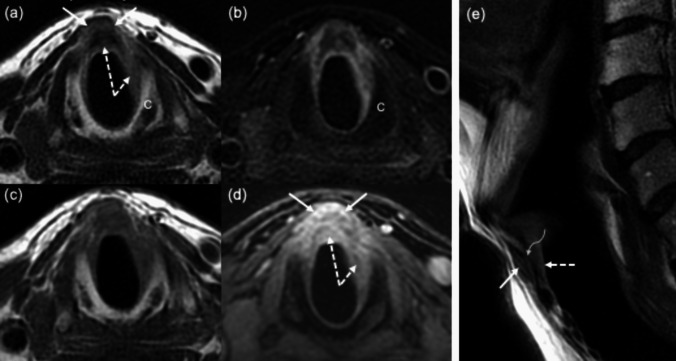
Subglottic level on axial FSE T2-weighted (a), FSE fat sat T2-weighted (b), FSE T1-weighted (c) and 3D gradient echo fat sat T1 post contrast (d); FSE T2-weighted on sagittal plane (e). A neoplastic submucosal thickening (white dashed arrows) with enhancement (d) in the anterior and left side of subglottic level. At the same level similar soft tissue representing extralaryngeal spread (white arrows) through the cricothyroid membrane (e) (curved arrow) is present. The cricoid cartilage (C) does not show abnormal alterations indicative of invasion. FSE, fast spin echo
